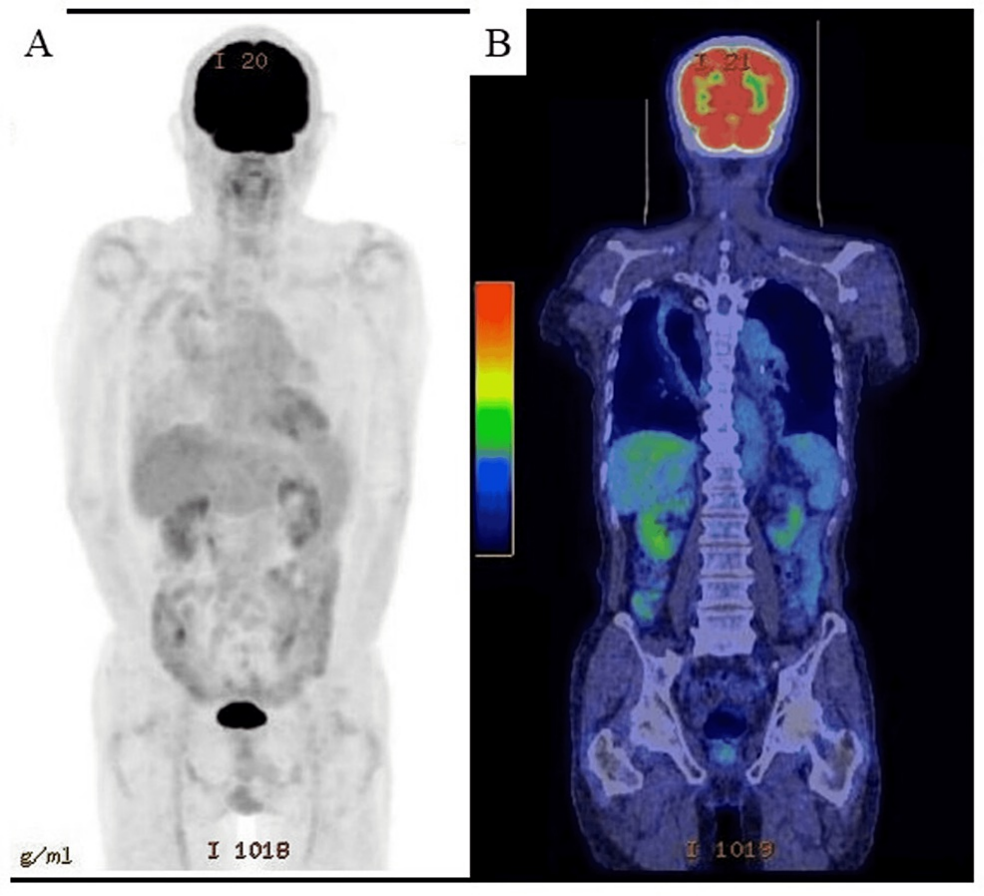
FDG positron emission tomography computed tomography at the time when brain tumor was detected. (A and B) The images show unremarkable FDG uptake in distant organs from the brain. FDG: fluorodeoxyglucose
